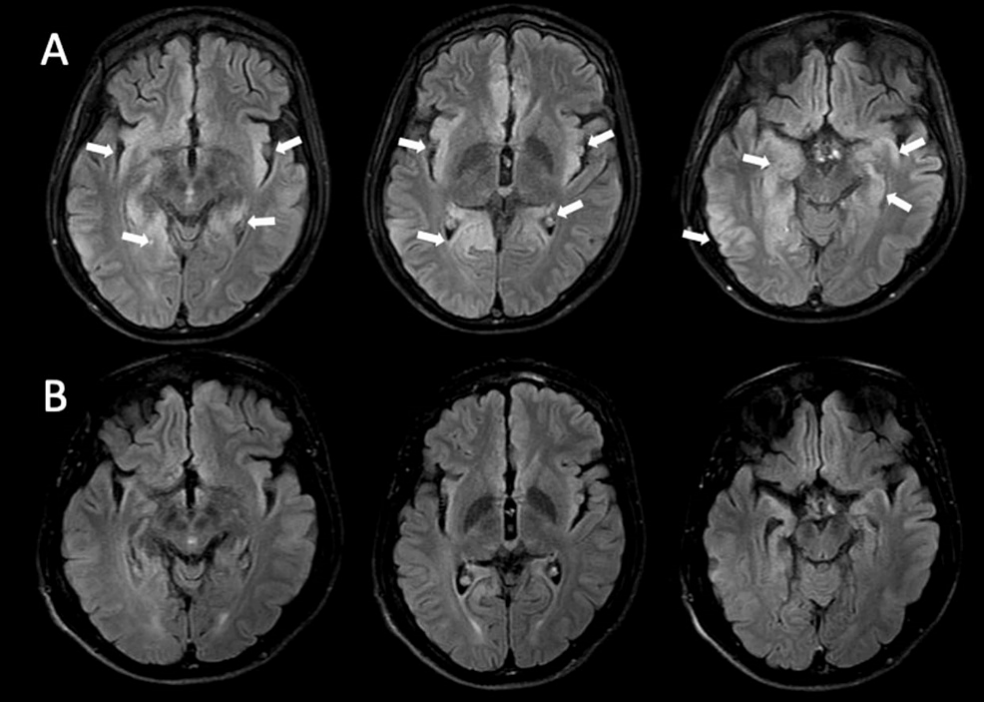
Hyperintense lesions observed in the axial FLAIR sequences, more prominent on the right side. (A) The lesions affect both cingulate gyri, bilateral insular cortices, amygdalae, hippocampi, and the right pre-cuneus. (B) After a three-month interval, axial FLAIR sequences demonstrated a substantial regression of the hyperintense lesions. FLAIR: fluid-attenuated inversion recovery
